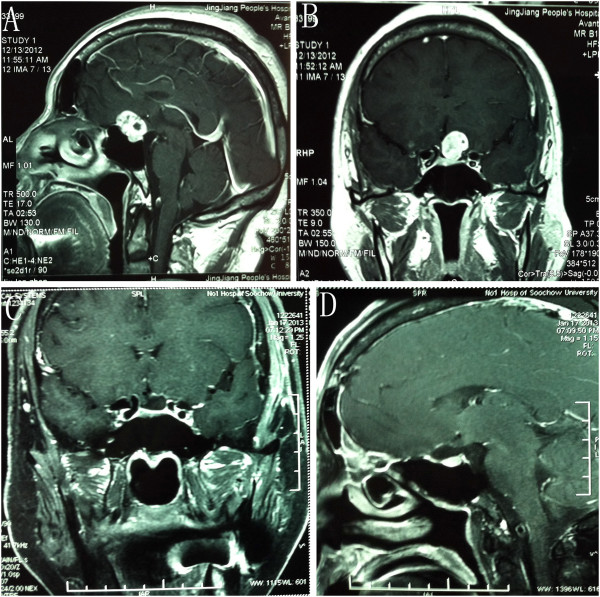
(A-B), preoperative MRI showing an intracranial optic nerve Schwannoma; (C-D) view of contrasted magnetic resonance imaging showing total removal of tumor.
